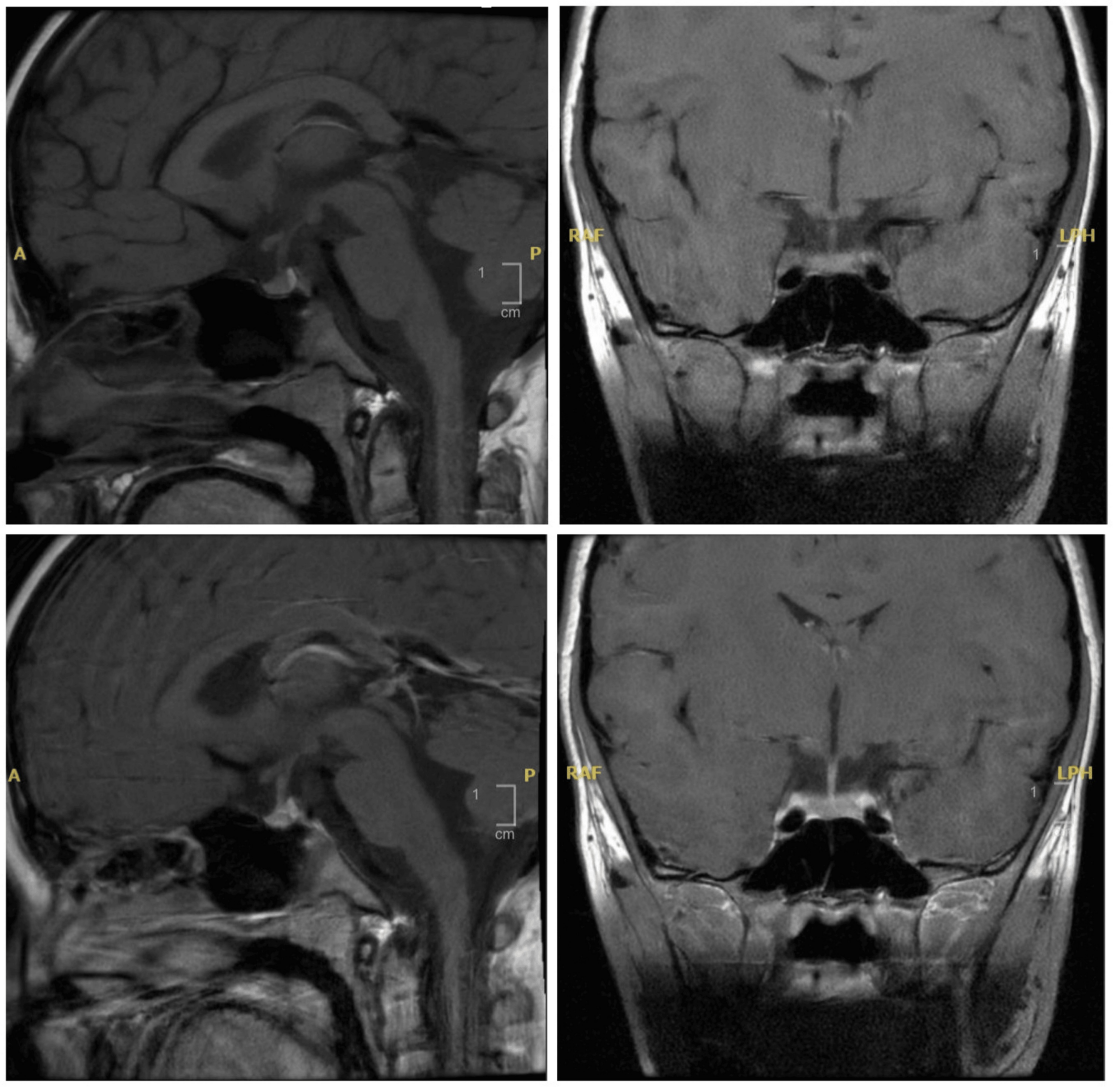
Pituitary MRI showed normal pituitary gland morphology and centralized pituitary stalk.
